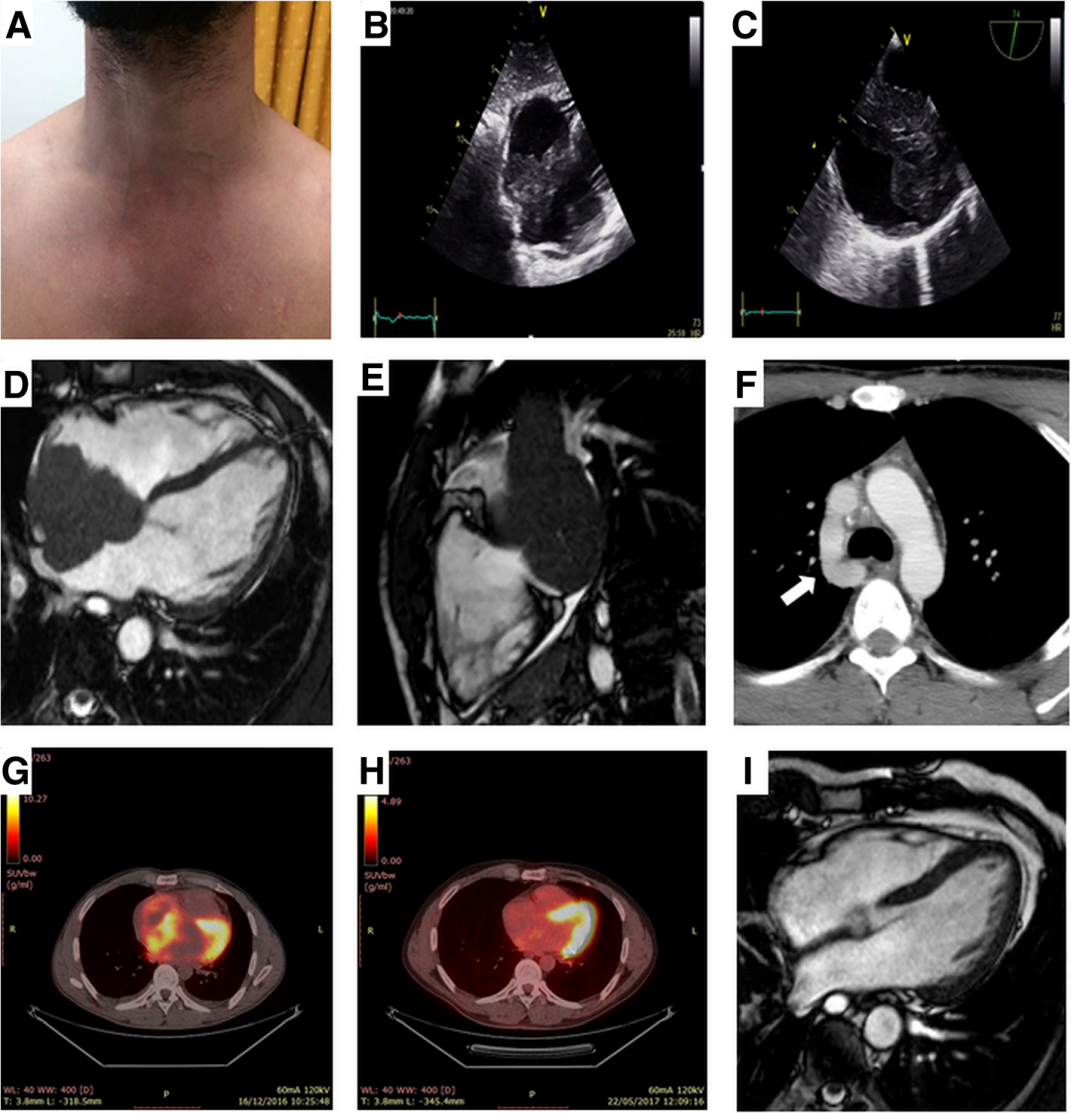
a. Dilated jugular veins and collaterals in the upper body. b. Transthoracic echocardiogram, subcostal view showing a large mass infiltrating the interatrial septum and extending mainly in the right atrium. c. Transesophageal echocardiogram, bicaval view showing a heterogeneous mass infiltrating the interatrial septum, filling almost three quarters of the right atrium, occluding the superior vena cava at its junction with the right atrium and extending into the left atrium. d. Cine MRI demonstrates a large infiltrating mass extending in both atria, occupying most of the right atrium, involving the surrounding pericardium leading to a mild pericardial effusion, and causing a grade of ostial stenosis of the lower right pulmonary vein. e. Cine MRI right ventricular long axis view with extension of neoplasm to the atriocaval junction and superior vena cava. f. Contrast-enhanced CT scan demonstrates a dilated azygos vein (arrow). g. 18-FDG PET/CT sagittal views showing increased 18-FDG-uptake within the tumor in the right atrium and h. complete tumor remission. i. Cine MRI four-chamber axial view showing no evidence of the tumor
